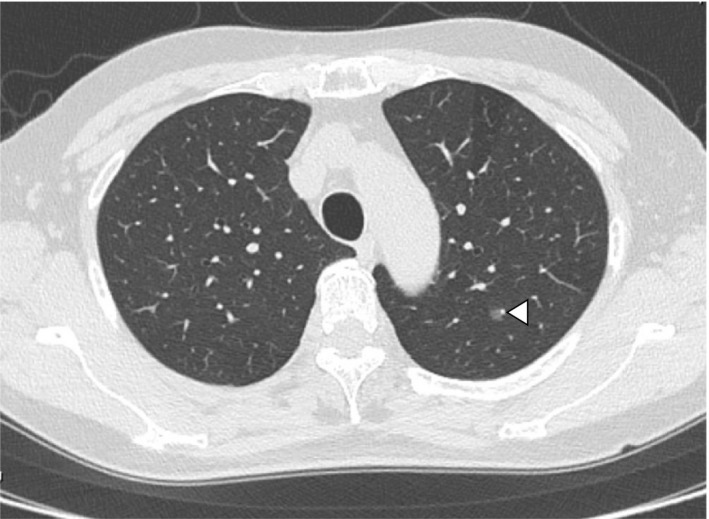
A CT image of the chest. An arrowhead indicates ground‐glass attenuation in the left lower lobe
